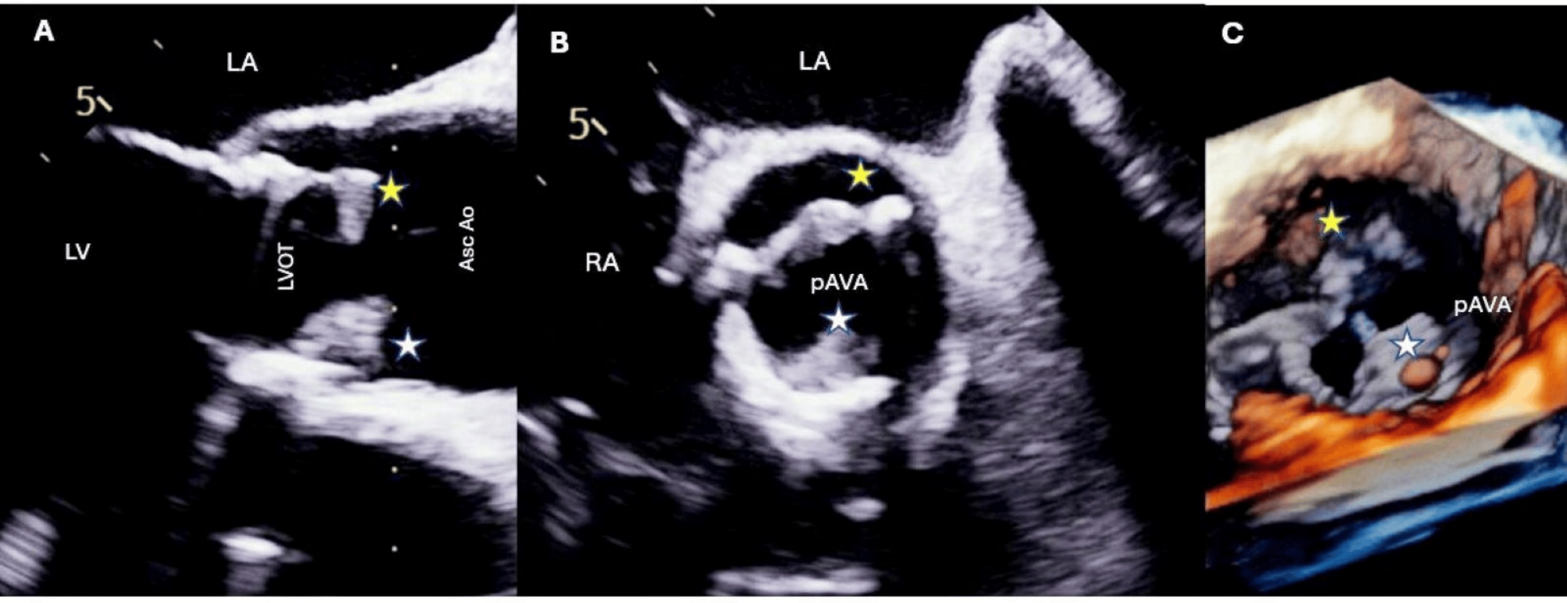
Transesophageal echocardiography revealed two mobile, irregularly shaped, echo-dense masses with independent oscillatory motion involving the anterior/right (yellow stars) and posterior/left (white stars) leaflets of the bioprosthetic aortic valve. The left panel (A) reflects the LVOT view at 120°, and the mid panel (B) reveals the short axis of the aortic valve view at 45–50%. The right panel (C) is a 3D image of the aortic valve demonstrating the bioprosthetic vegetations. LA: left atrium; LV: left ventricle; LVOT: left ventricle outflow tract; Asc. Ao: ascending aorta; pAV: prosthetic aortic valve
